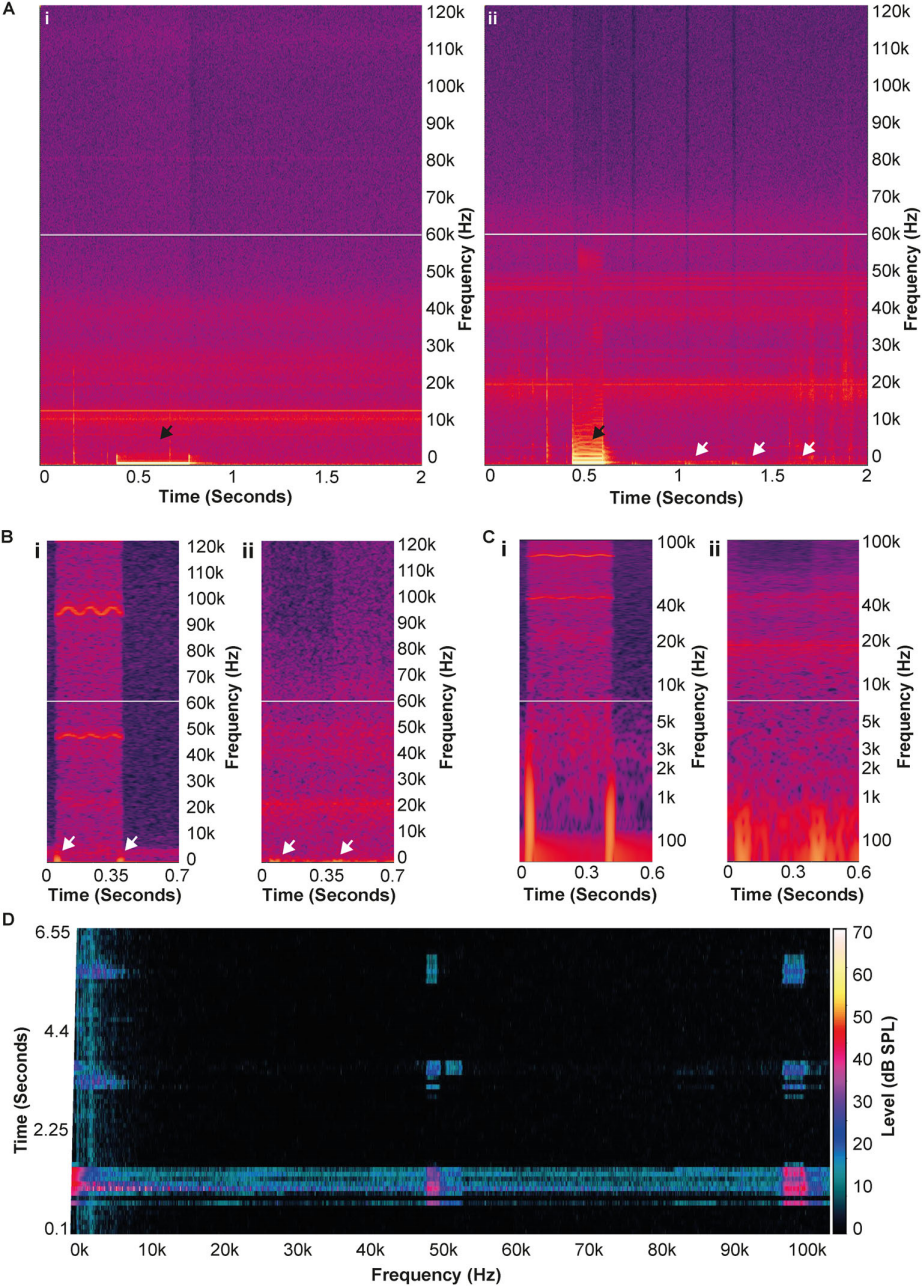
The walkie-talkie noise was likely radio frequencies (RF) interference. A) Spectrograms of the AudioMoth recordings of the radio being used in an isolated room (i), and in close proximity to an active monitor speaker (ii). The black arrows denote the audible beep produced when the transmit button was pressed. The white arrows in (ii) show the RF interference picked up by the active monitor speaker, while the absence of these in (i) shows that the noise did not arise from the walkie-talkie alone. B) Comparison of the spectrograms from the Avisoft recording (i) and the AudioMoth recording of the radio by the speaker (ii). The white arrows again denote the audible clicks in both recordings, which are not produced by the walkie-talkie itself (as shown in [Ai]); note the ultrasonic noise picked up the Avisoft system are bordered by these clicks. C) The same comparison as (B) using a logarithmic frequency scale to highlight the temporal similarities between the noise from the Avisoft system and the radio being operated in close proximity to the speaker. D) FFT spectrogram using the PULSE software to analyse the recording made by the Avisoft system. Noise can clearly be seen at the same frequencies that were originally detected by the Avisoft system, indicating that if the noise was not an artefact, the PULSE system would have also detected it.
